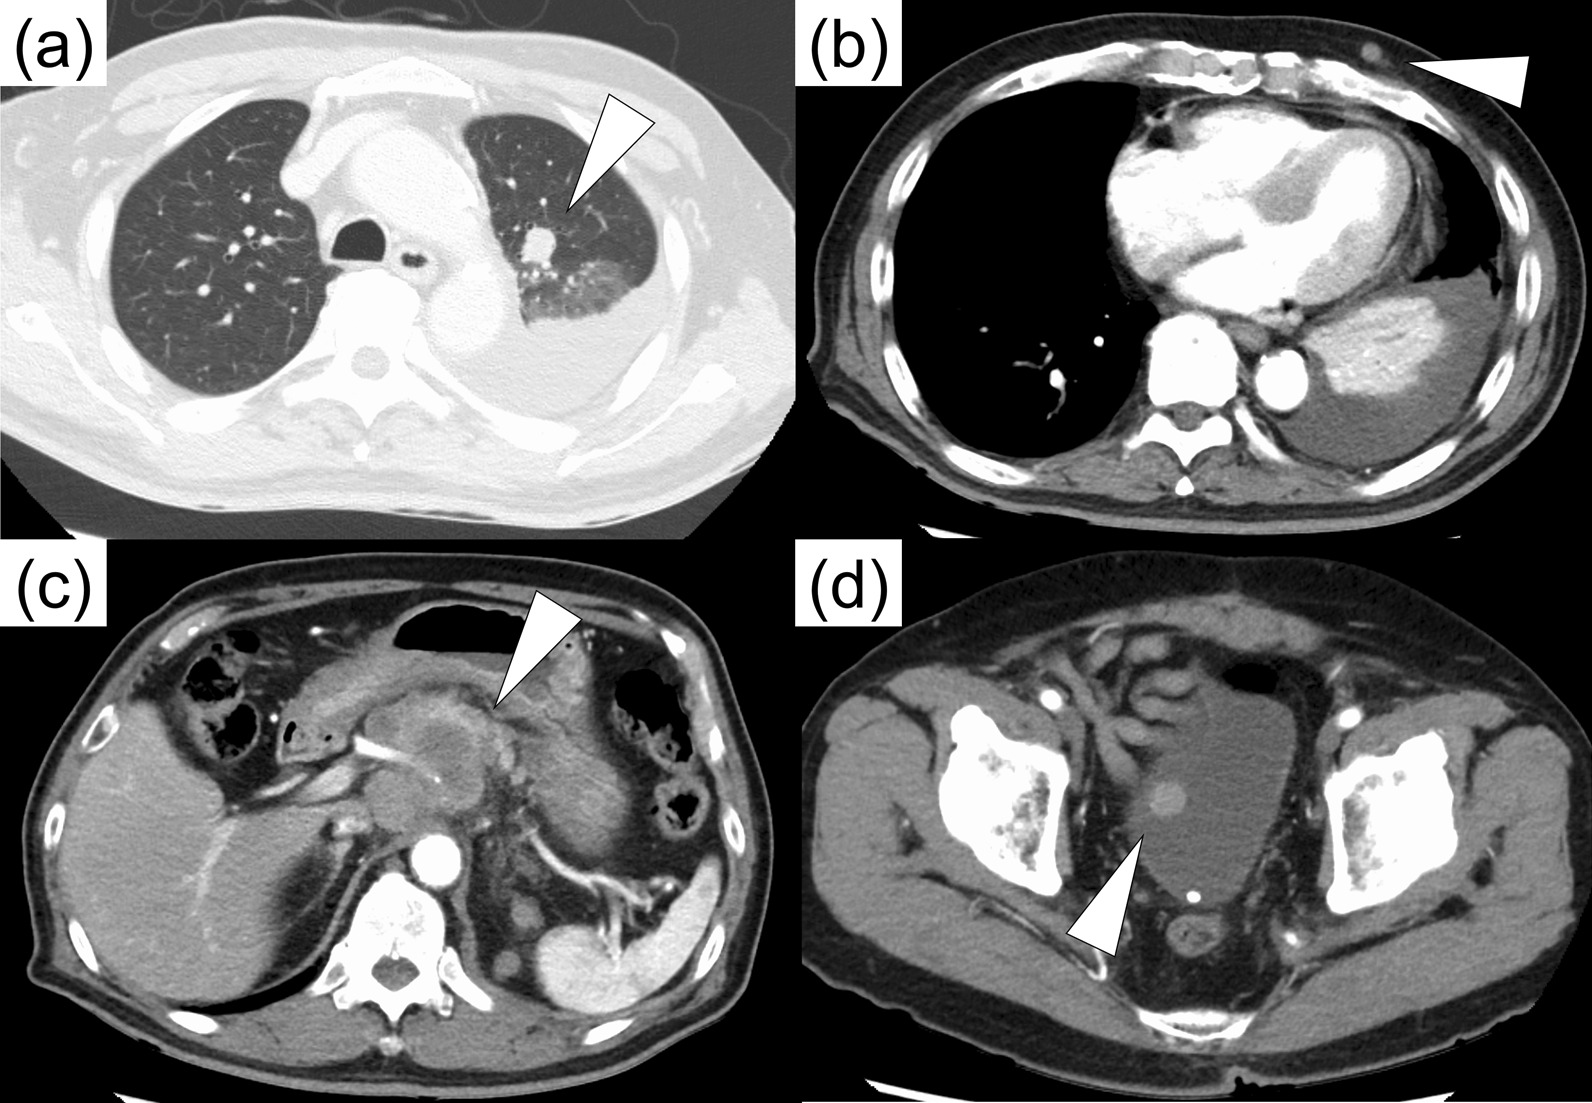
Contrast computed tomography revealed tumors (white arrowheads) in the left lung (a), subcutaneous tissue (b), abdominal lymph nodes (c), and bladder (d) consistent with metastatic disease
